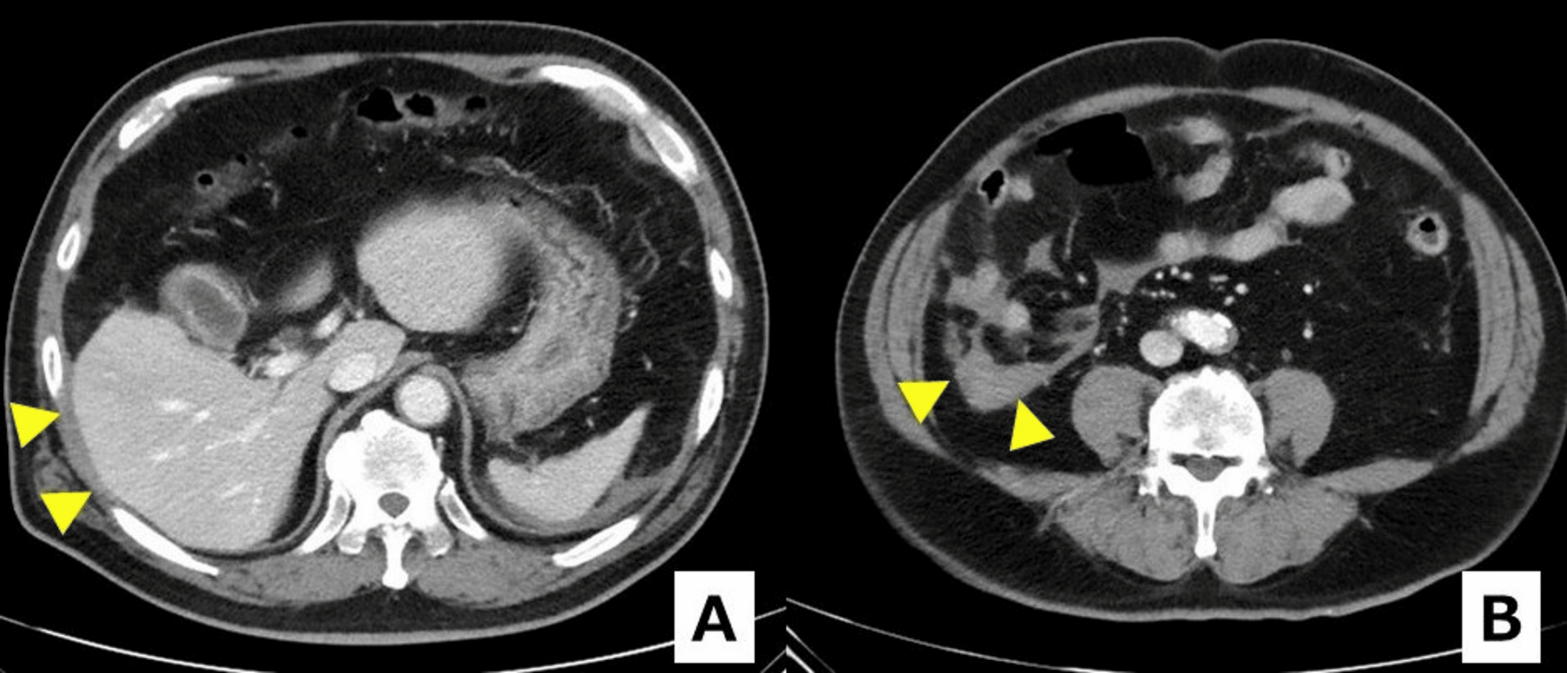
Follow-up contrast-enhanced CT one week after tube dislodgement (A and B) Arrowheads indicate residual bloody ascites that has decreased in volume compared with the prior scan but persists along perihepatic and right paracolic spaces. The attenuation remains above simple fluid (~0-20 HU) and compatible with acute blood (~30-45 HU), again supporting hemoperitoneum, with no mass-like hematoma or organized collection. CT: computed tomography, HU: Hounsfield units
